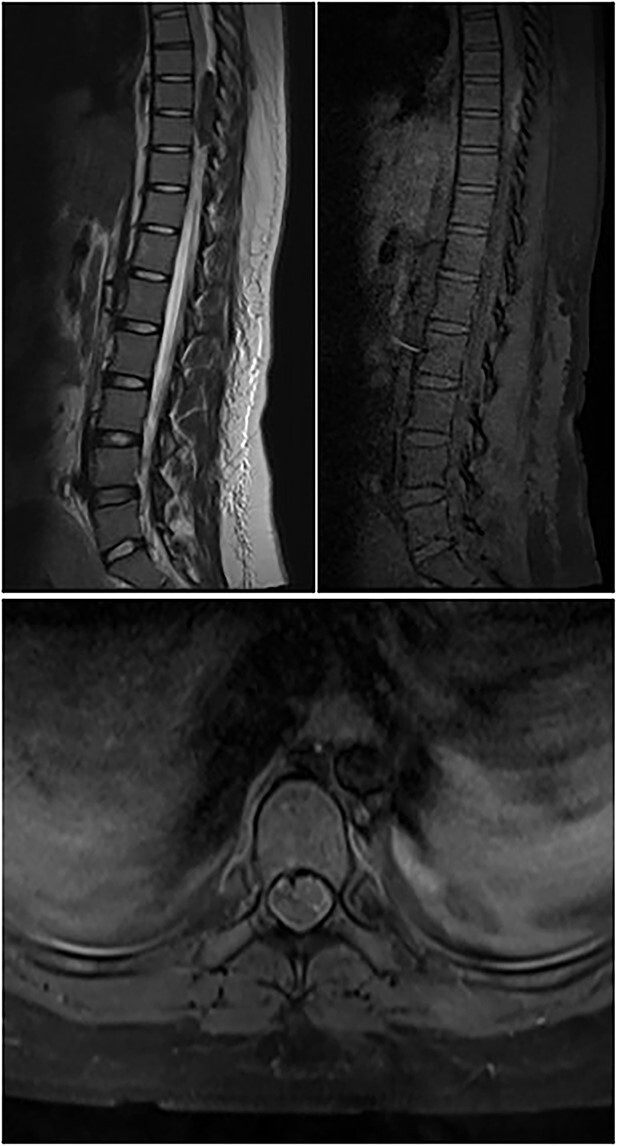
Top left image shows preoperative T2W sagittal MRI of the dorsal spine showing hypointense lesion in the posterior epidural space at D8–9; top right image shows T1W pre-contrast fat-suppressed image showing hyperintensity; bottom image shows post-contrast T1W axial image showing minimal contrast enhancement.
